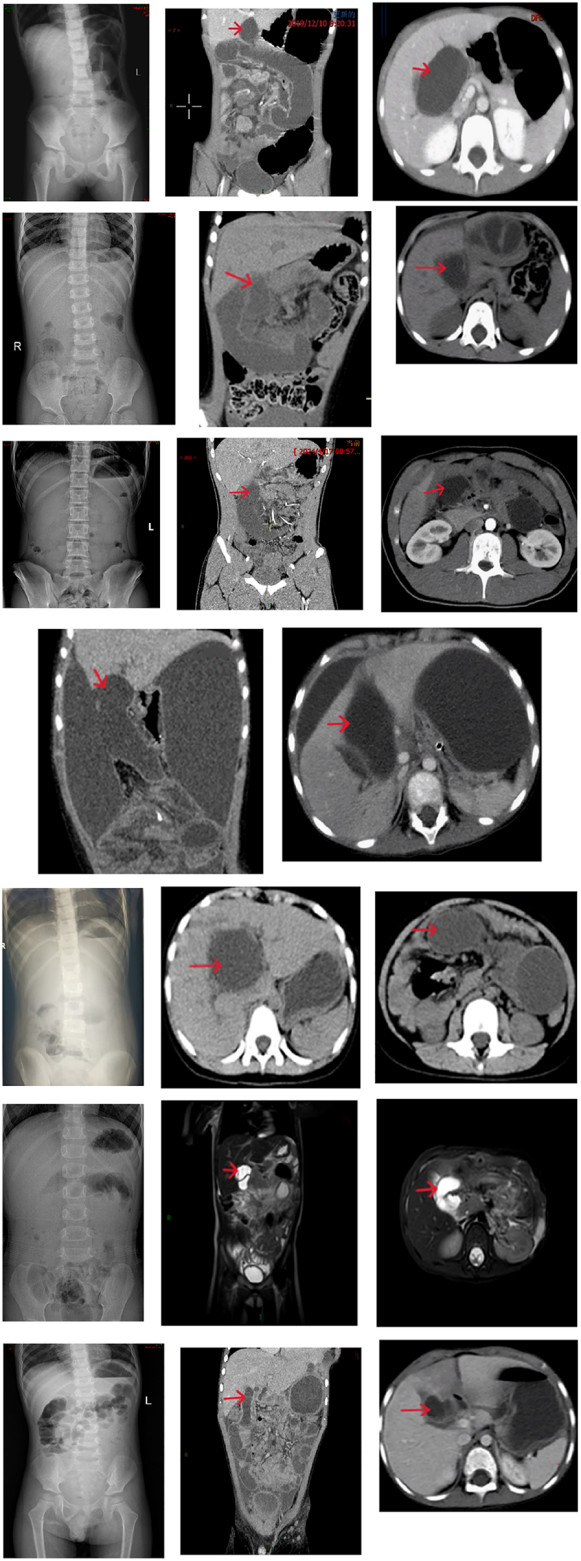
Case 1: The bile duct Y-loop is twisted 720°, appearing as a cord-like structure. The arrow indicates the dilated bile duct loop at the first hepatic hilum. Case 3: Transverse mesocolic defect hernia (small intestine herniates through the mesocolic defect, with the herniated segment located 130 cm from the cecum). The arrow indicates the dilated bile duct loop at the first hepatic hilum. Case 5: Petersen's hernia (proximal jejunum, including the jejunojejunal anastomosis, herniates into the Petersen's space causing a 720° rotation of the bile duct loop and necrosis of the bile duct loop). The arrow indicates the dilated bile duct loop at the first hepatic hilum. Case 6: Brolin hernia (the bile duct loop herniates into the Brolin's space, with complete necrosis of the biliary-jejunal loop and the biliary-jejunal loop jejunojejunal anastomosis). The patient did not undergo an abdominal x-ray preoperatively. The arrow indicates the dilated bile duct loop at the first hepatic hilum. Case 7: Transverse colonic mesenteric defect hernia (the proximal jejunum, including the jejunojejunal anastomosis, herniates into the colonic mesenteric defect, resulting in necrosis of the bile duct loop). This patient underwent intestinal obstruction surgery at a medical alliance hospital and no coronal CT images were available. The arrow indicates the dilated bile duct loop at the first hepatic hilum. Case 8: Petersen's hernia (herniation of the intestinal segment, 120 cm from the cecum, into the Petersen's space). The patient did not undergo abdominal CT examination. The arrow indicates the dilated bile duct loop at the first hepatic hilum. Case 9: Colonic mesenteric defect hernia (herniation of the intestinal segment, 100 cm from the cecum, into the mesocolic defect of the transverse colon). The arrow indicates the dilated bile duct loop at the first hepatic hilum.
